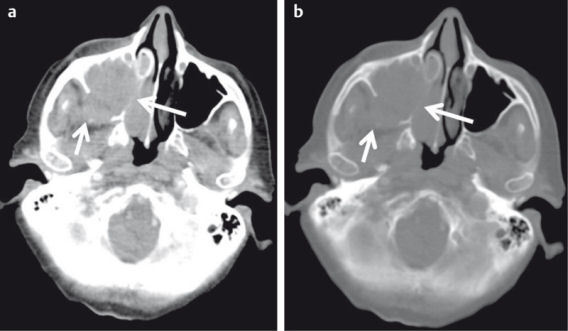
Contrast-enhanced CT of the midface with squamous cell carcinoma of the right maxillary sinus. a) Axial image in bone window level setting. Complete opacification of the right maxillary sinus with tumor extension to the retromaxillary fossa (short arrow) and the nasal cavity. Infiltration of the medial wall of the maxillary sinus. b) Infiltration of small bony structures can better be appreciated in bone window-level settings.
